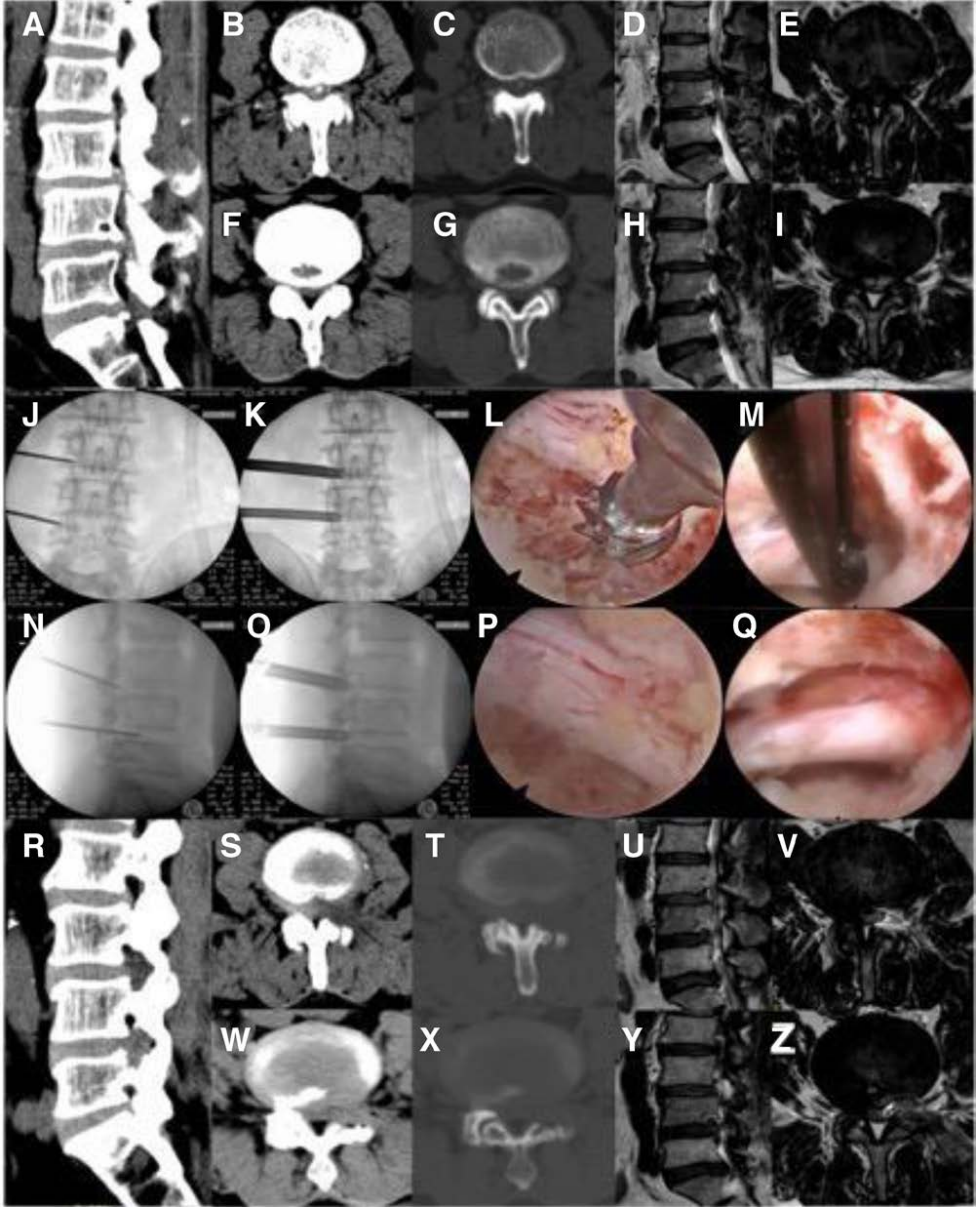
(A) patient diagnosed with double level LSS with ossification experienced double level unilateral PETD surgery. (A–I) Preoperative magnetic resonance imaging and computed tomography show intervertebral disc calcification in L3/4 and posterior edge separation in L4/5. (R–Z) Postoperative magnetic resonance imaging and computed tomography show that double level ventral part of the SAP, prominent nucleus pulposus and ventral ossification of dural sac and nerve root have been removed. The lateral recess and neuroforamen are enlarged. (J–Q) Superior arthroplasty was performed simultaneously on both levels. Endoscopy showed that the nerve roots were fully decompressed in L3/4 (L, M) and L4/5 (P, Q).
